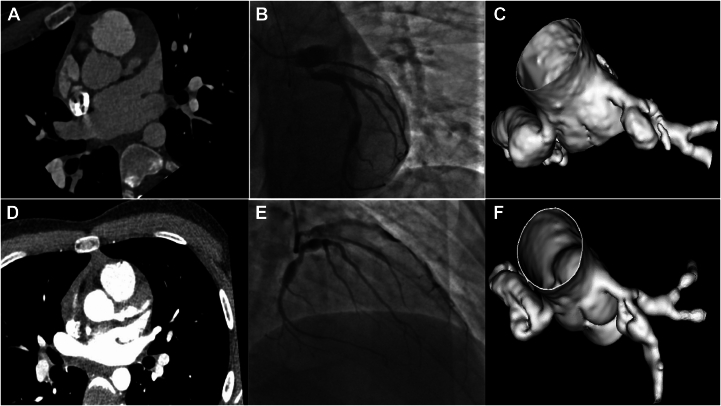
LAD before intervention. CCTA images (A) and 3D reconstruction (C) showed proximal occlusion of aneurysmal LAD, which was corroborated on angiography (B). Postangioplasty angiography demonstrated restoration of flow to the distal LAD (E). CCTA images (D) and 3D reconstruction (F) 48 hours later showed continued vessel patency. CCTA, coronary computed tomography angiogram; LAD, left anterior descending coronary artery
